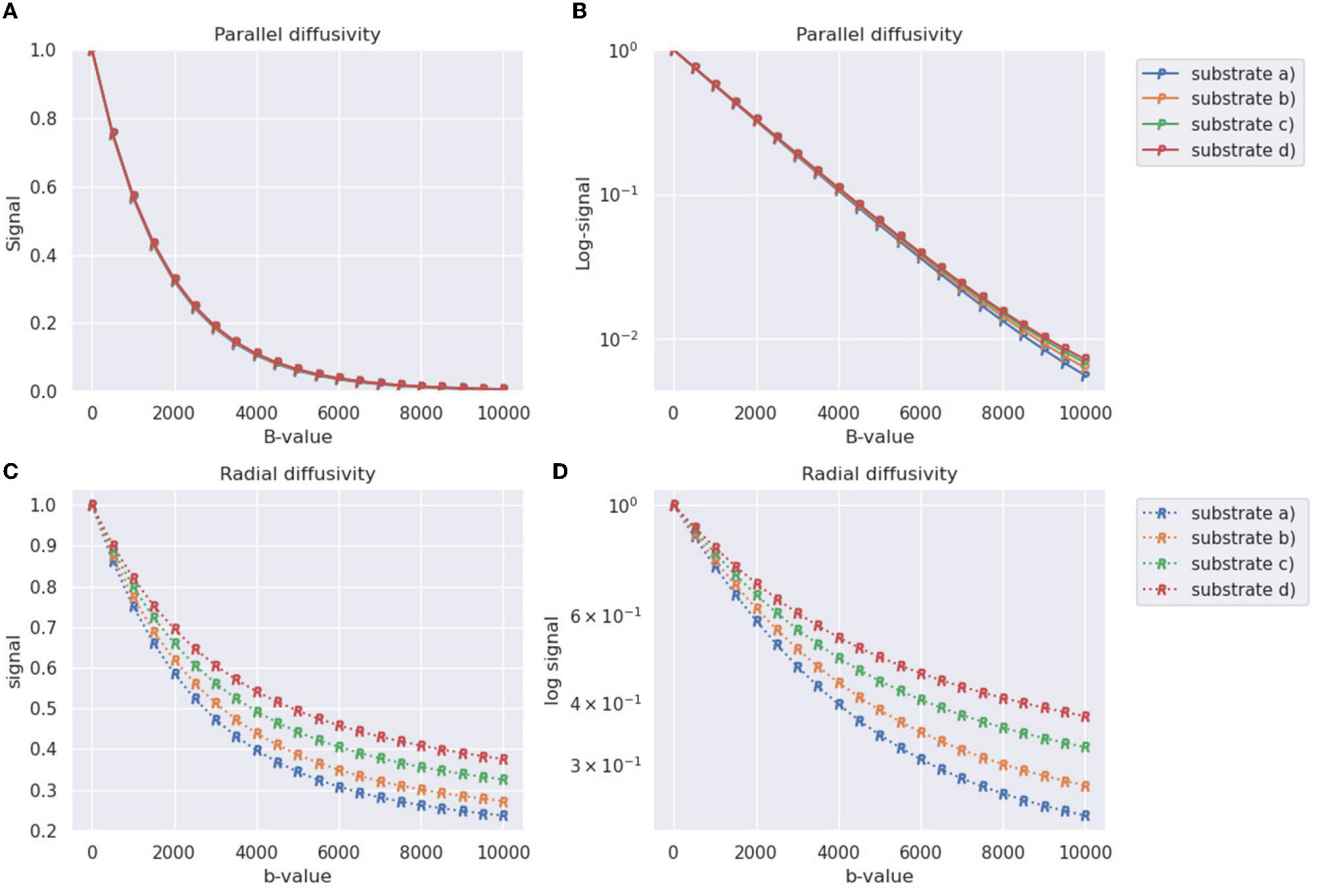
Synthetic DW-MRI signals of substrates generated in Table 2 and shown in Figure 8. The X-axis represents the b-value used for measurement, while the Y-axis represents the normalized signal. (A) Parallel signal decay measured along the direction parallel to the substrate fibers (Z-axis). (B) Parallel signal decay plot with a logarithmic scale on the Y-axis. (C) Radial signal decay averaged over four different diffusion directions orthogonal to the orientation of the fibers. (D) Radial signal decay plot with a logarithmic scale on the Y-axis.
